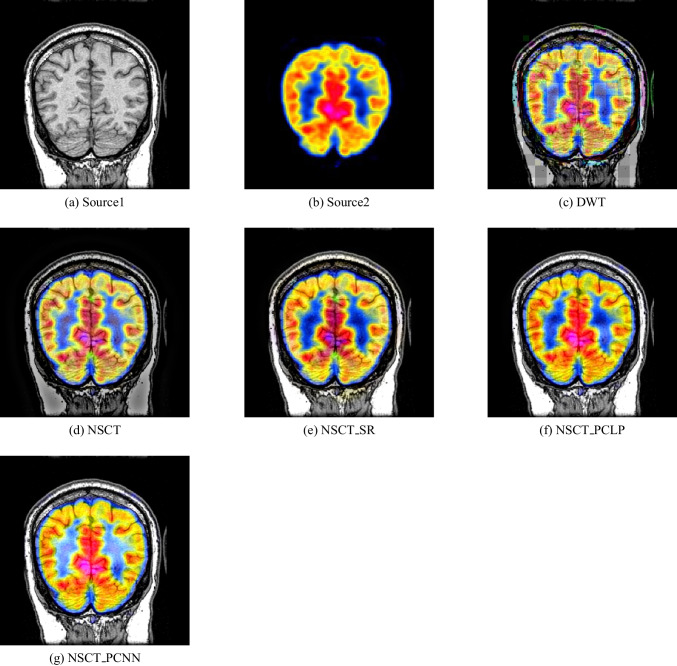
Fusion result of (a) MR-T2 and (b) SPECT using (c) DWT method, (d) NSCT method, (e) NSCT-SR method, (f) NSCT-PCLP method, and the proposed method (g) NSCT-PCNN
